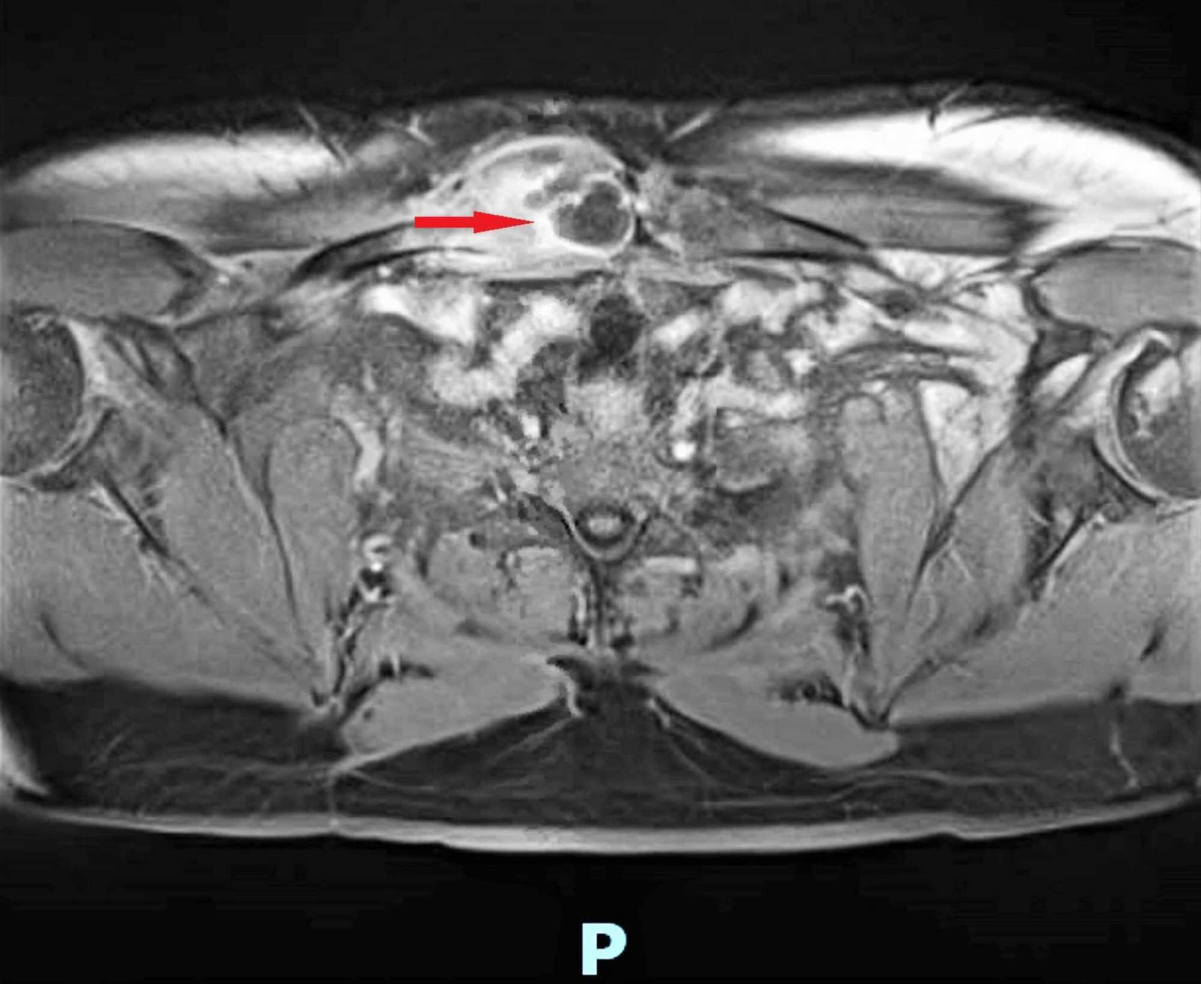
MRI of the right sternoclavicular joint showing right-sided sternoclavicular septic arthritis and osteomyelitis with associated periarticular abscess (red arrow) MRI: magnetic resonance imaging
